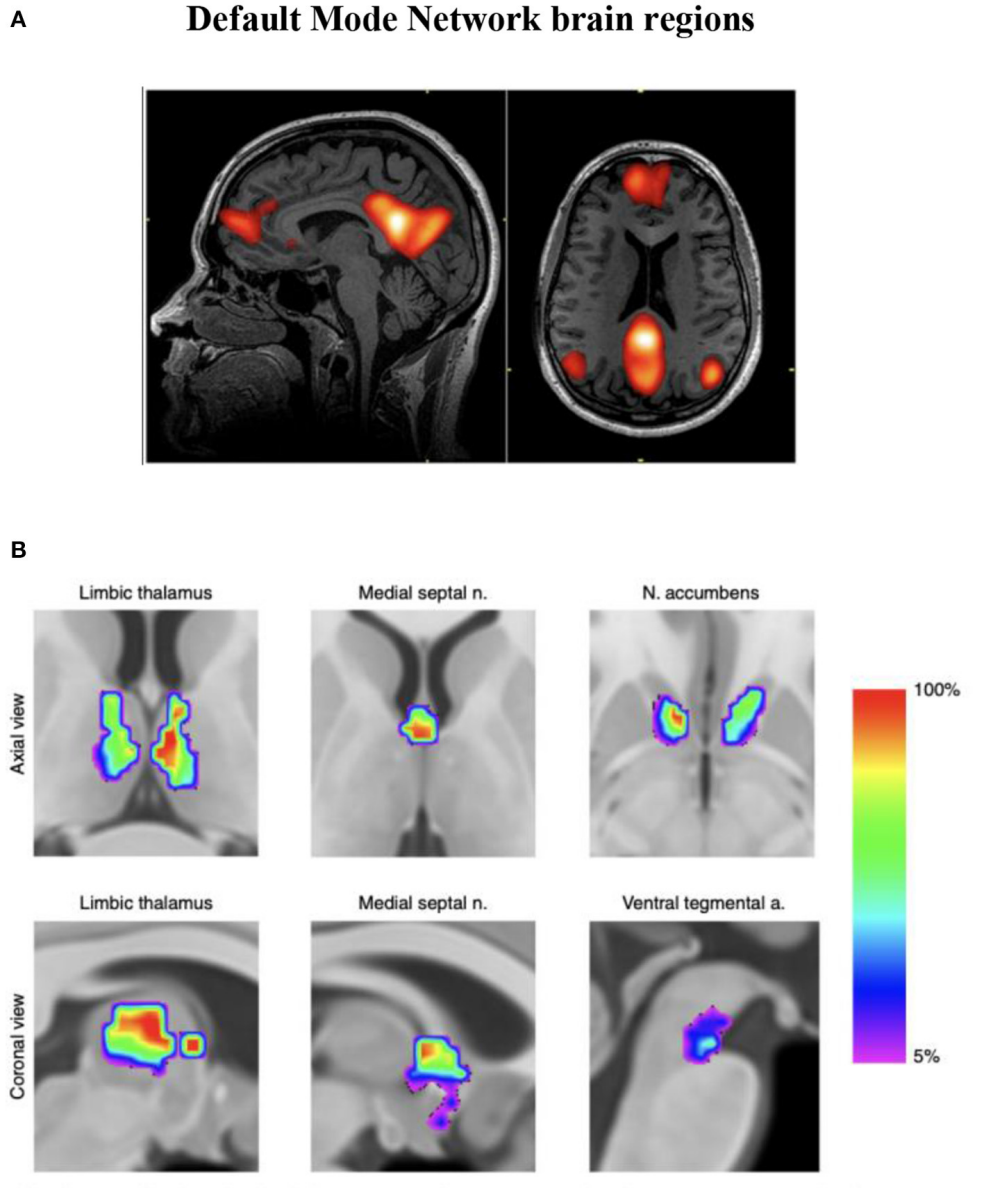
(A) Functional MRI demonstration of the default mode network (DMN). (B) Discrete neuroanatomical model of the DMN showing coactivation of brainstem regions. This map is freely available at https://www.nature.com/articles/s42003-019-0611-3/figures/4. n = 20 participants. Improved neuroanatomical model of the default-mode network shows co-activation of brainstem and midbrain regions with DMN hubs. This reconciles previous neuroimaging and neuropathological findings (Alves et al., 2019).
